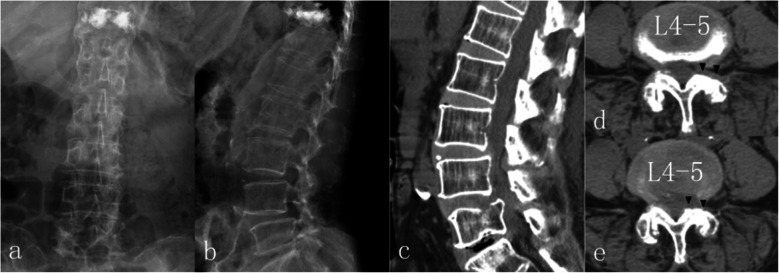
DR 3 days postoperatively demonstrated no slippage deterioration at L4–5 level (a-b). Postoperative CT re-examination detected anterior portion of superior articularis of L4 was removed, as well as the partial of L4–5 disc (c-e: triangle)
